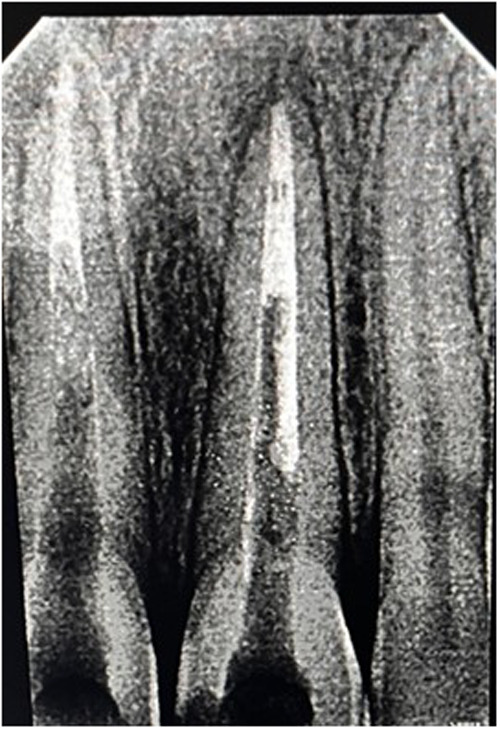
IOPA radiographic representation of orthograde obturation of pulp space with MTA.
